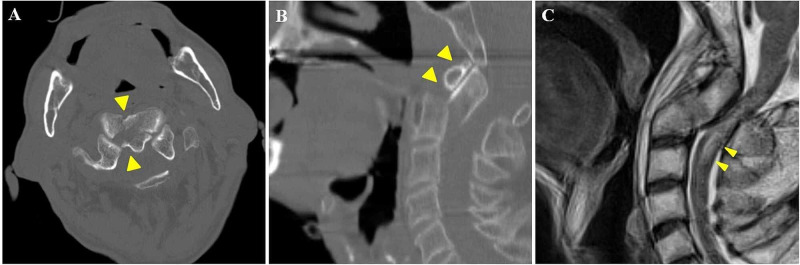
(A) CT revealed a fracture of the C2 vertebra (arrowhead). (B) Sagittal reconstruction of the CT images revealed a posteriorly displaced bone fragment (arrowhead). (C) Sagittal T2-weighted MRI of the neck showed a hyper-intensity area from C1 to C2 (arrowhead).
